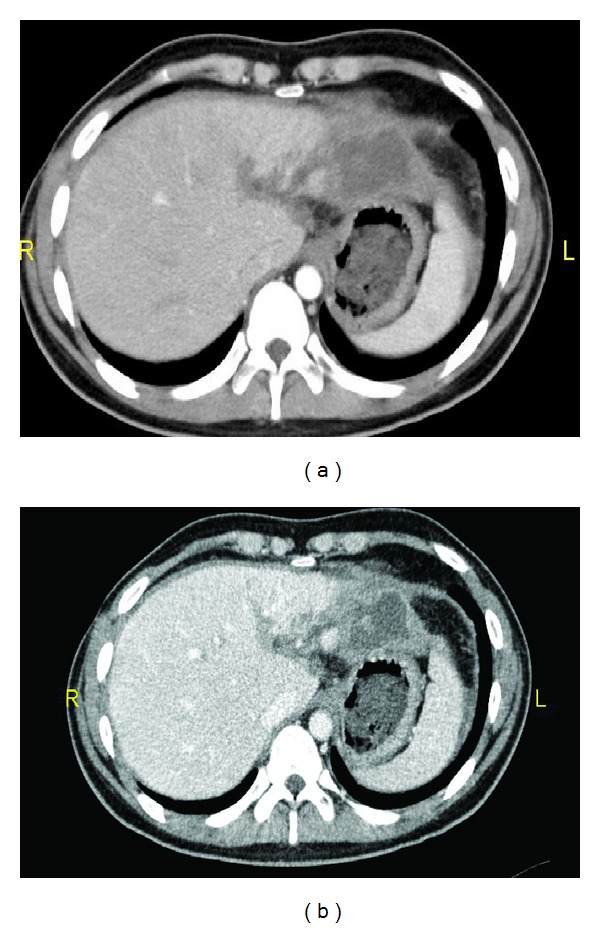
Follow-up abdomen CT (one year): (a) regeneration of right lobe and (b) shrunken left lobe.
